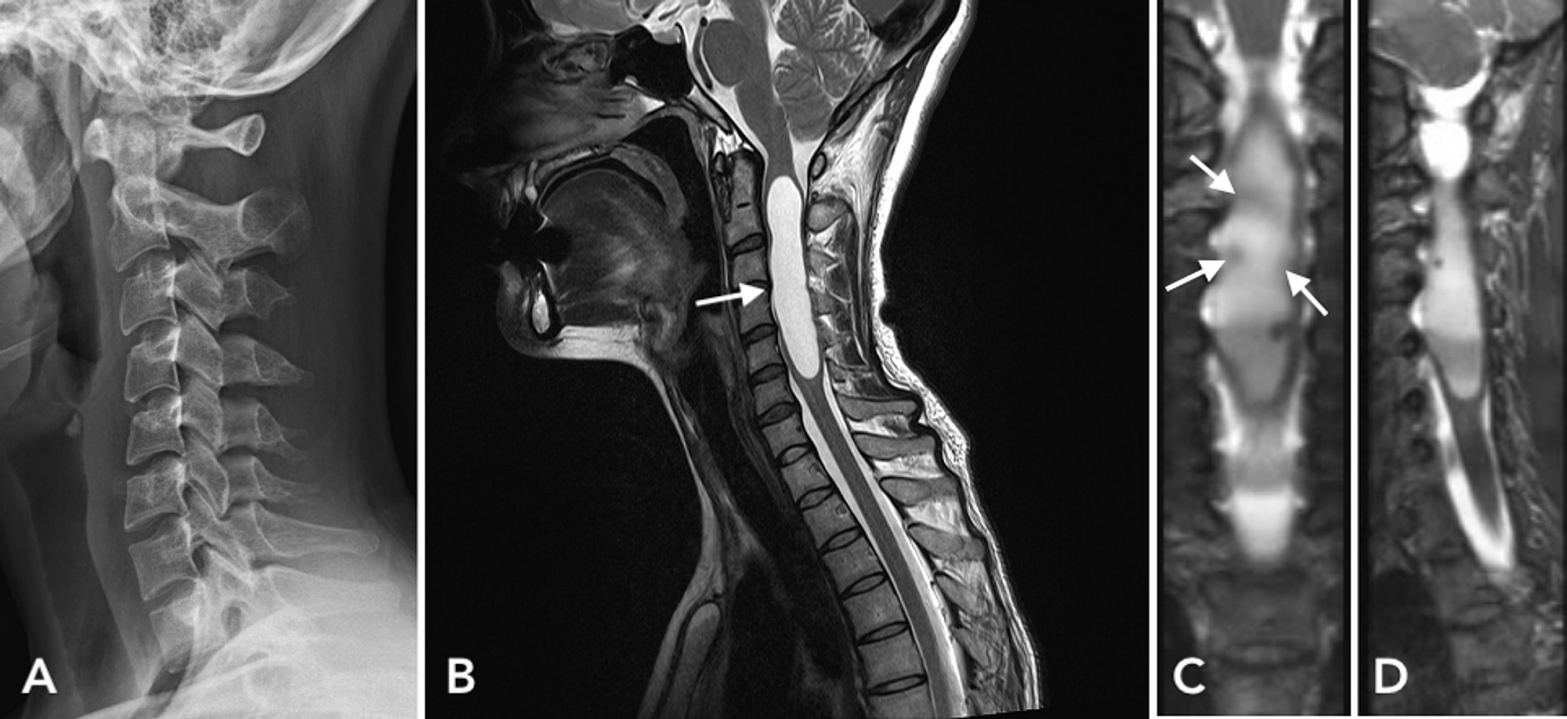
Cervical X-ray showing widening of the spinal canal between C3-C5 (A). Sagittal spinal MRI showing large syrinx that extends from C2 to C6 (B). T2 weighted image (T2WI)-MRI coronal view shows multiple mural nodules with some contrast enhancement (arrows) (C), sagittal view (D)
